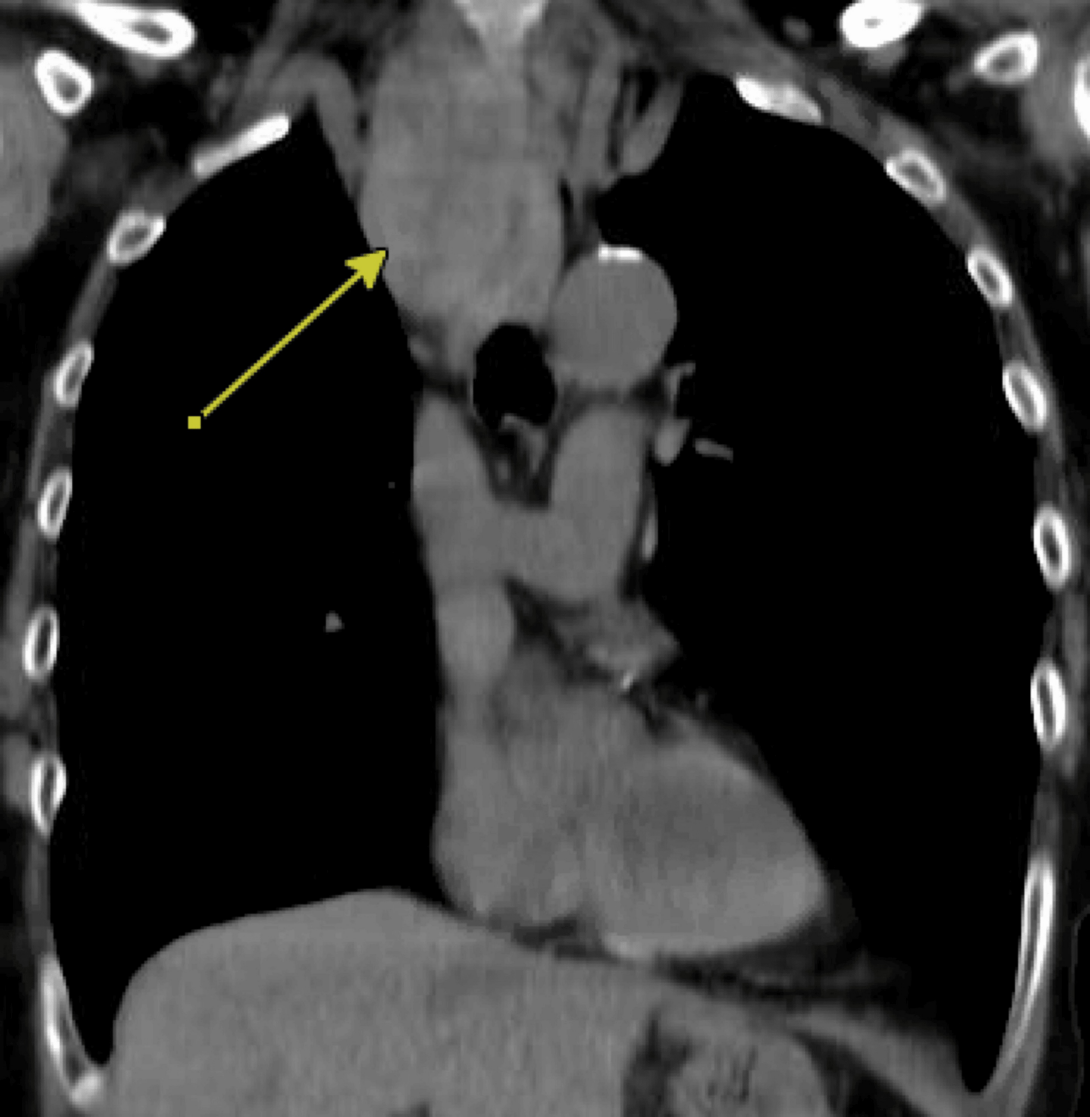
Case 1: CT scan of the chest Arrow pointing to the mediastinal goiter
